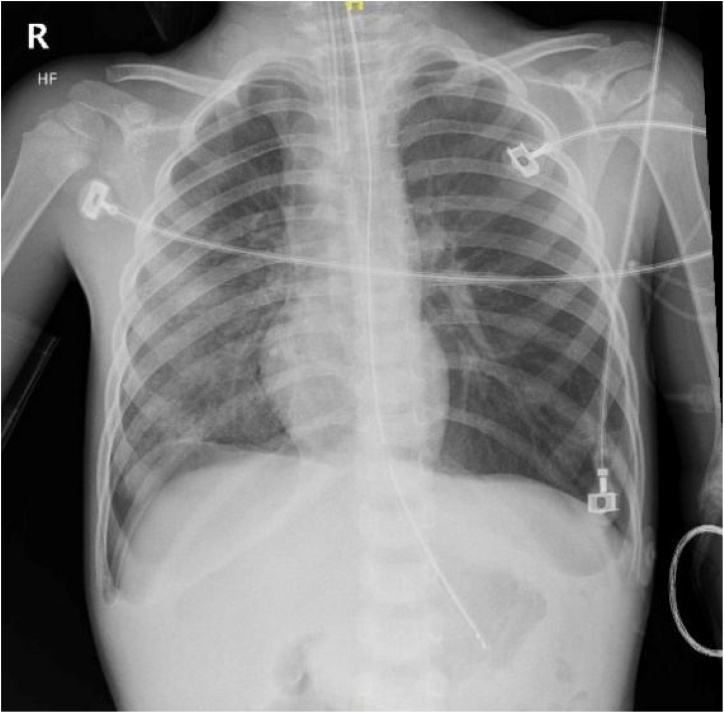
Post-Operative Day 1 Chest radiograph.
